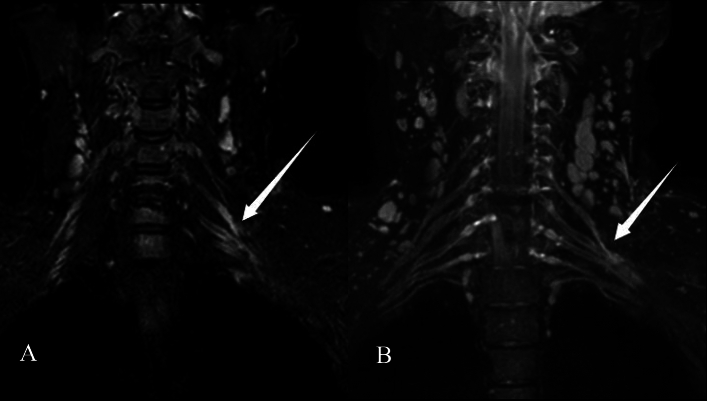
A 49-year-old woman with left-sided symptoms who underwent brachial plexus MRI following neck trauma. On the left C5 posterior ganglion and upper trunk, marked hyperintensity on coronal 3D SHINKEI sequence (A) and reconstructed MIP image (B) has been recorded (arrows). Surgery confirmed negative. This case has been considered a false positive.
